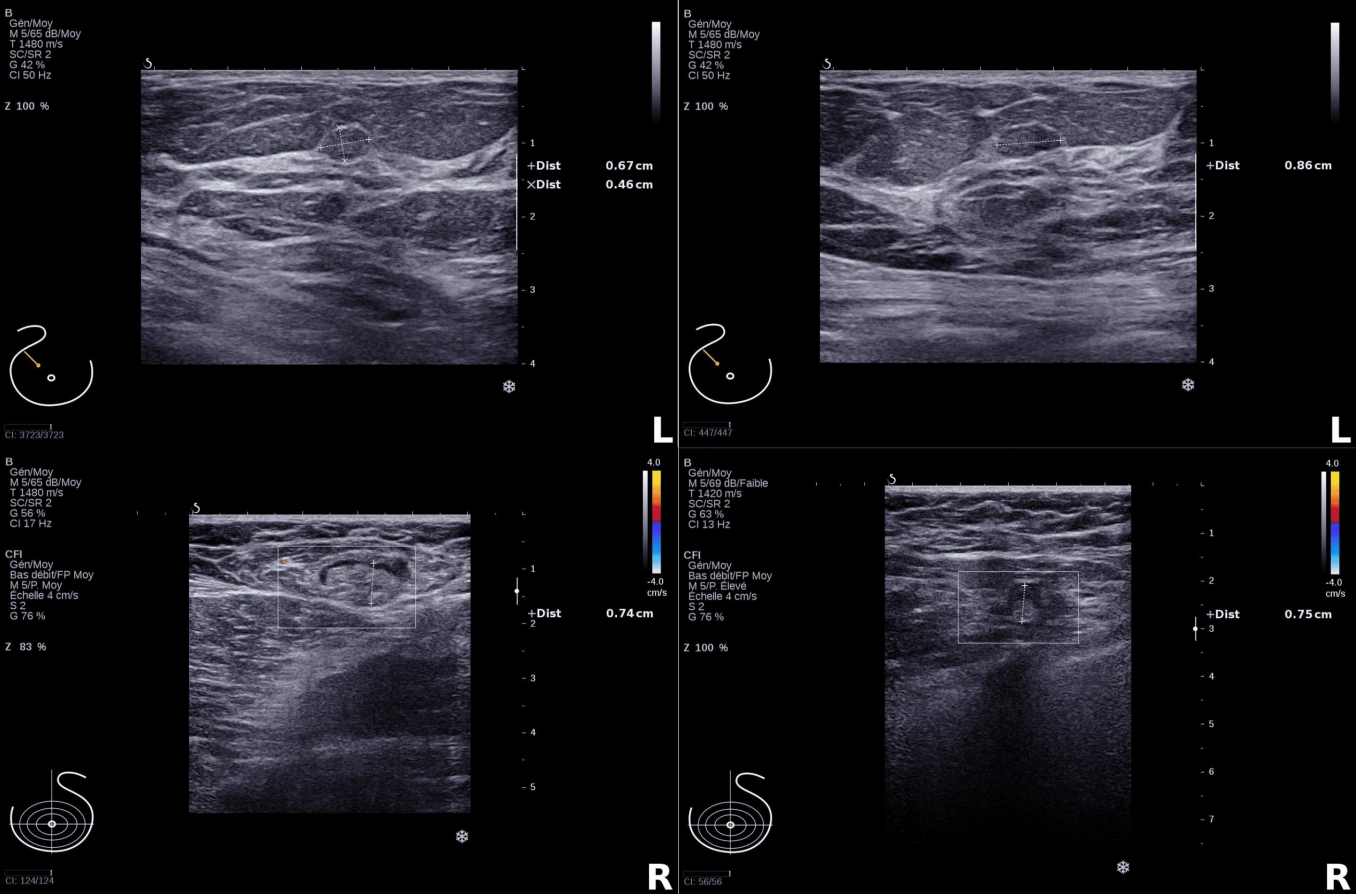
Ultrasonography of the breasts. Upper panels: transverse views of the left breast showing two well-defined, hypoechoic nodules (L). Lower panels: transverse views of the right breast showing two avascular, benign-appearing oval structures (R).
